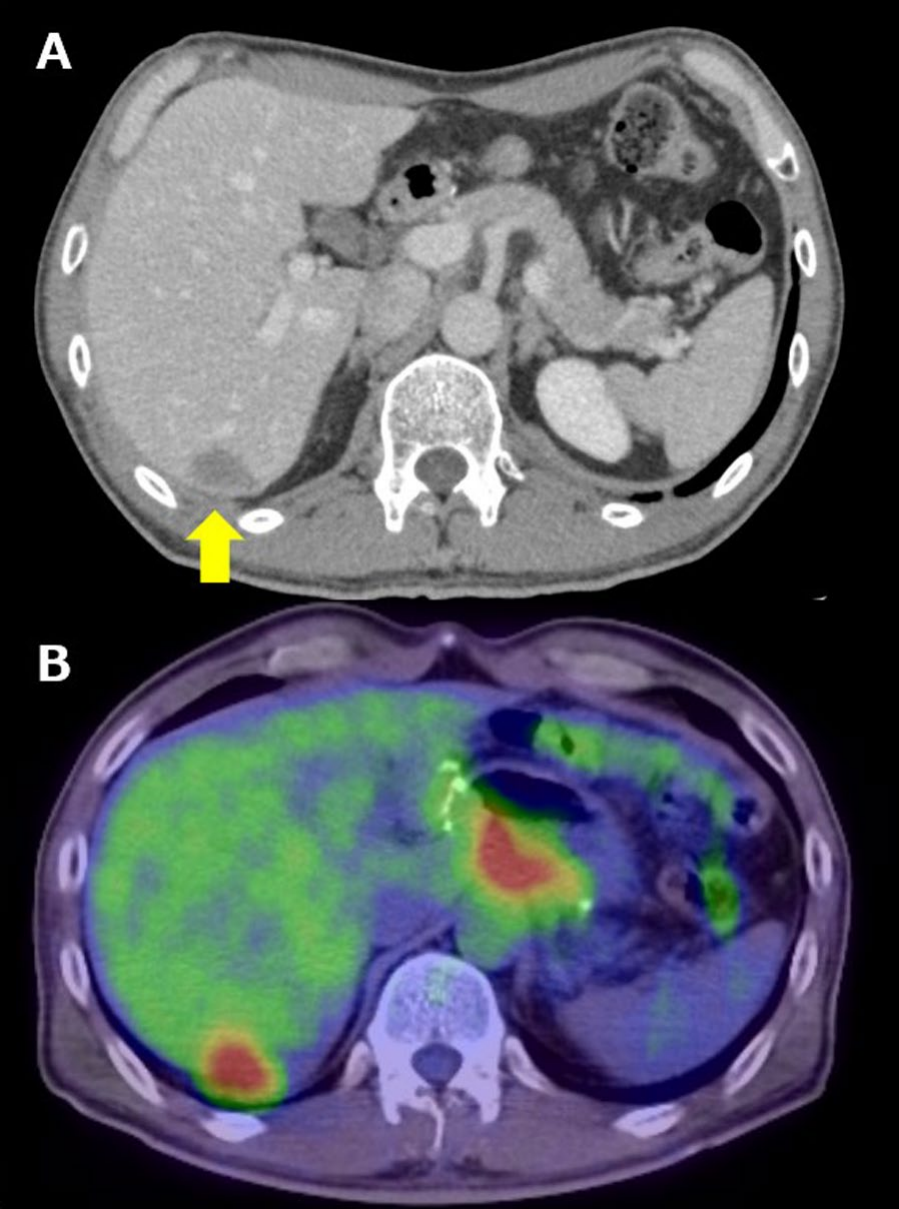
Result of CT 1 year after initial surgery. A CT showing a 2.5 cm low-density area in segments 6 and 7 of the liver. B FDG–PET performed at the same time as CT showing abnormal uptake at the site of liver metastasis
